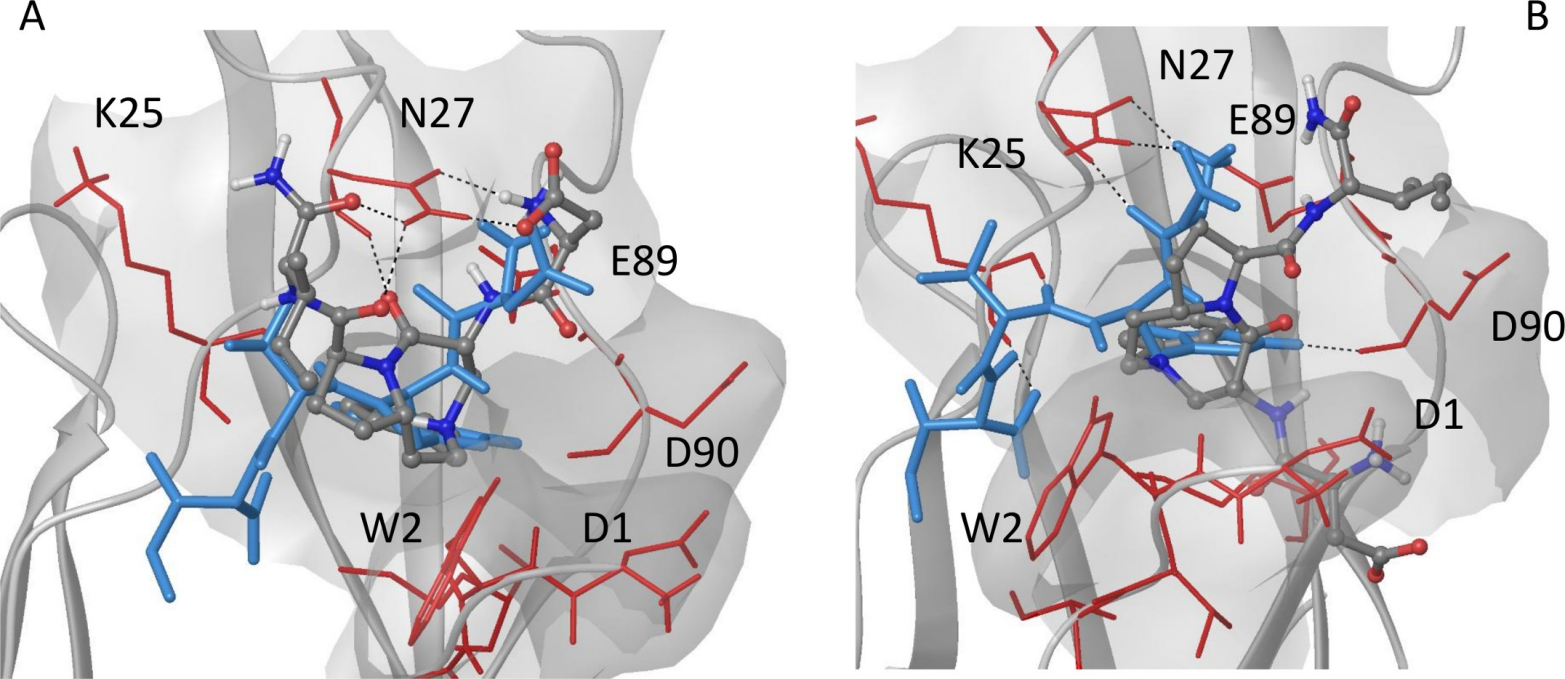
Docking preferred binding modes of compound 2 into E-cadherin. Type A is shown for the ligand charged state (left) and type B for the ligand neutral state. The compound (grey carbon atoms) is displayed into the E-cadherin X-ray structure (PDB code: 3Q2V) superimposed to the DWVI sequence (light blue). Residues of the binding pocket interacting with the ligand are labelled.
